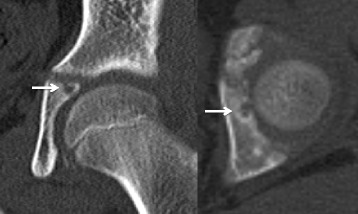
Patient computed tomography images on admission. Coronal view (*left*) and axial view (*right*) of computed tomography examinations showing a well-demarcated 5 mm mass surrounded by bone sclerosis (*arrows*) in the left acetabulum adjacent to the triradiate cartilage
